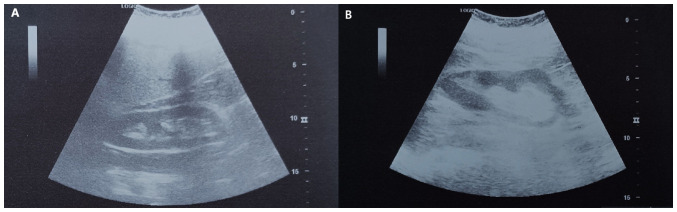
Post-operative ultrasound examination of the urinary system, revealing normal kidney morphology without the pre-operative dilatation of the pyelocaliceal system and the presence of the perinephric urinoma; (A) right kidney, (B) left kidney.
